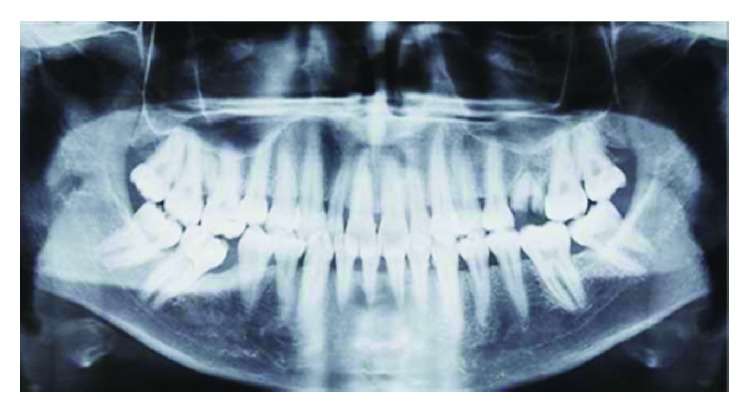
Baseline radiographic examination.
